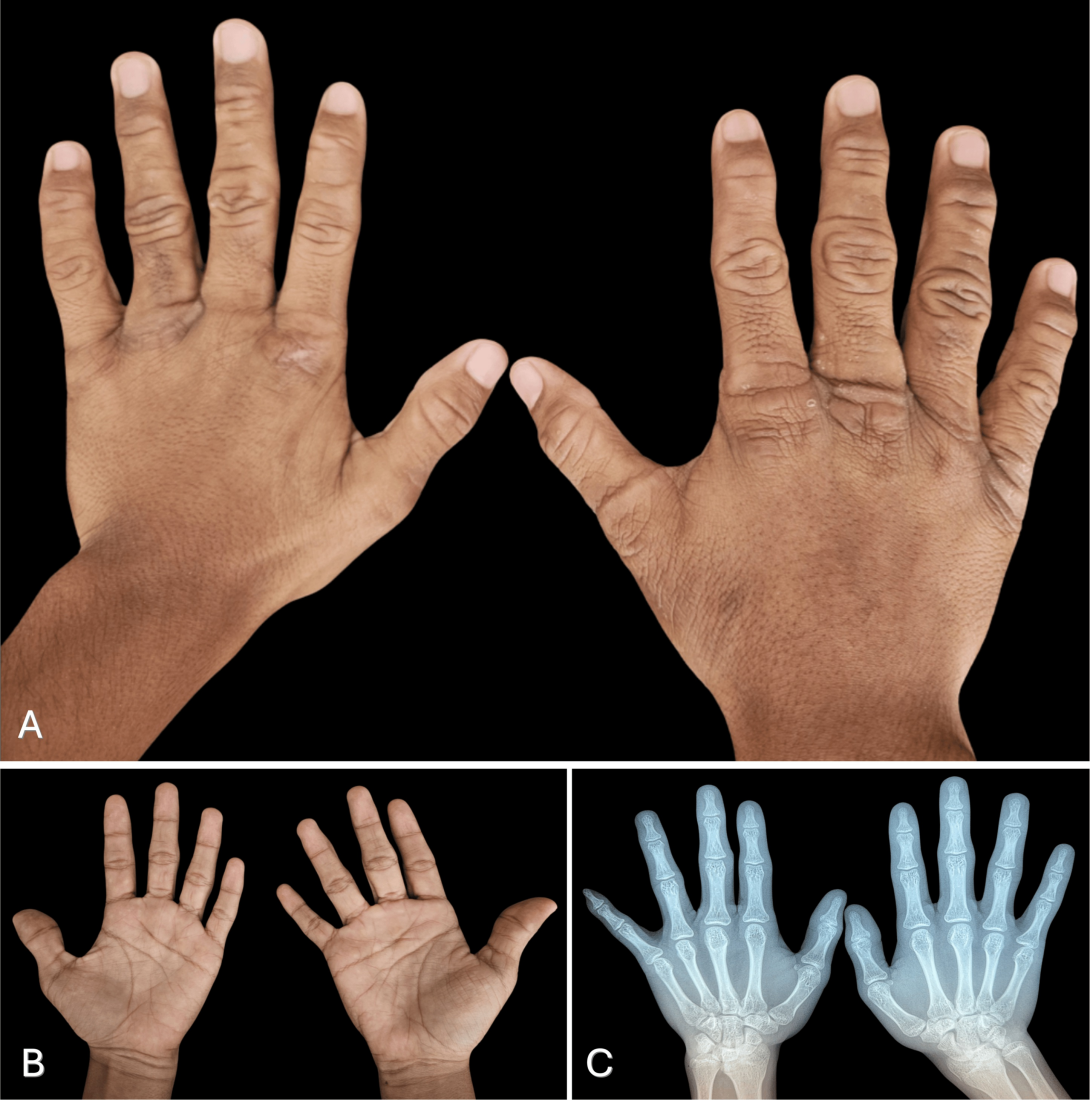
Clinical image and X-ray of hands (A-B) Involvement of the dorsal region of the hands, with a predominance in the interphalangeal region consisting of thickening, hyperpigmentation, and hyperlianity. (C) Radiographs demonstrated increased soft tissue volume without evidence of bone involvement
